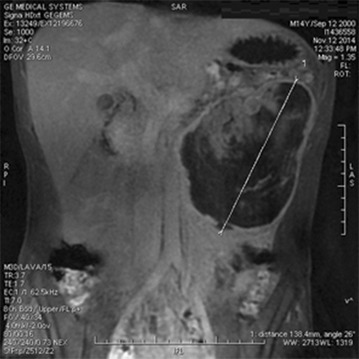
Magnetic resonance imaging (MRI) of the abdominal mass
